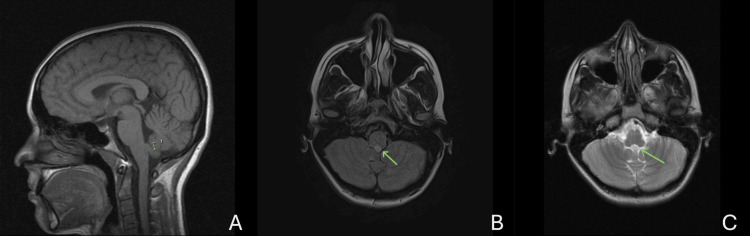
Six-month follow-up MRI demonstrating radiographic stability A. Sagittal T1-weighted image showing unchanged size and location of the fourth-ventricular lesion (bracket). B. Axial postcontrast T1-weighted sequence showing no interval enhancement or mass effect from the lesion (arrow). C. Axial T2-weighted postcontrast image demonstrating stable signal characteristics without new obstruction or edema (arrow).
